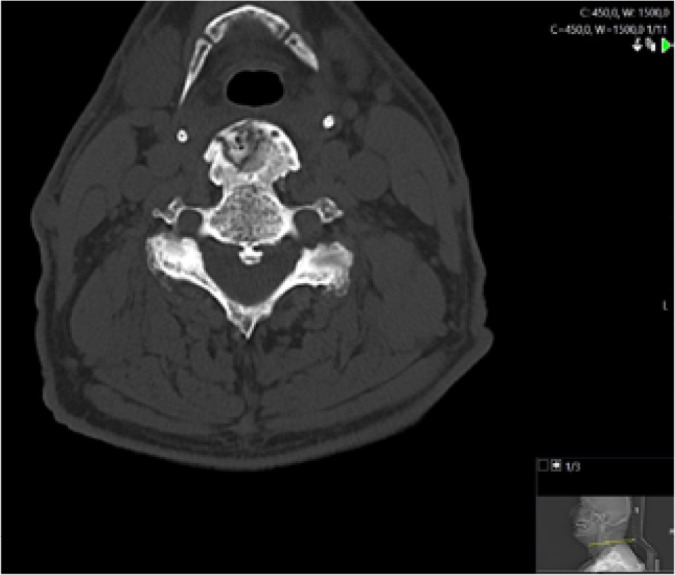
Axial CT scan at the C3-C4 level reveals prominent anterior ossification in close proximity to the laryngopharynx. At this level, calcification of the posterior longitudinal ligament is also observed.
